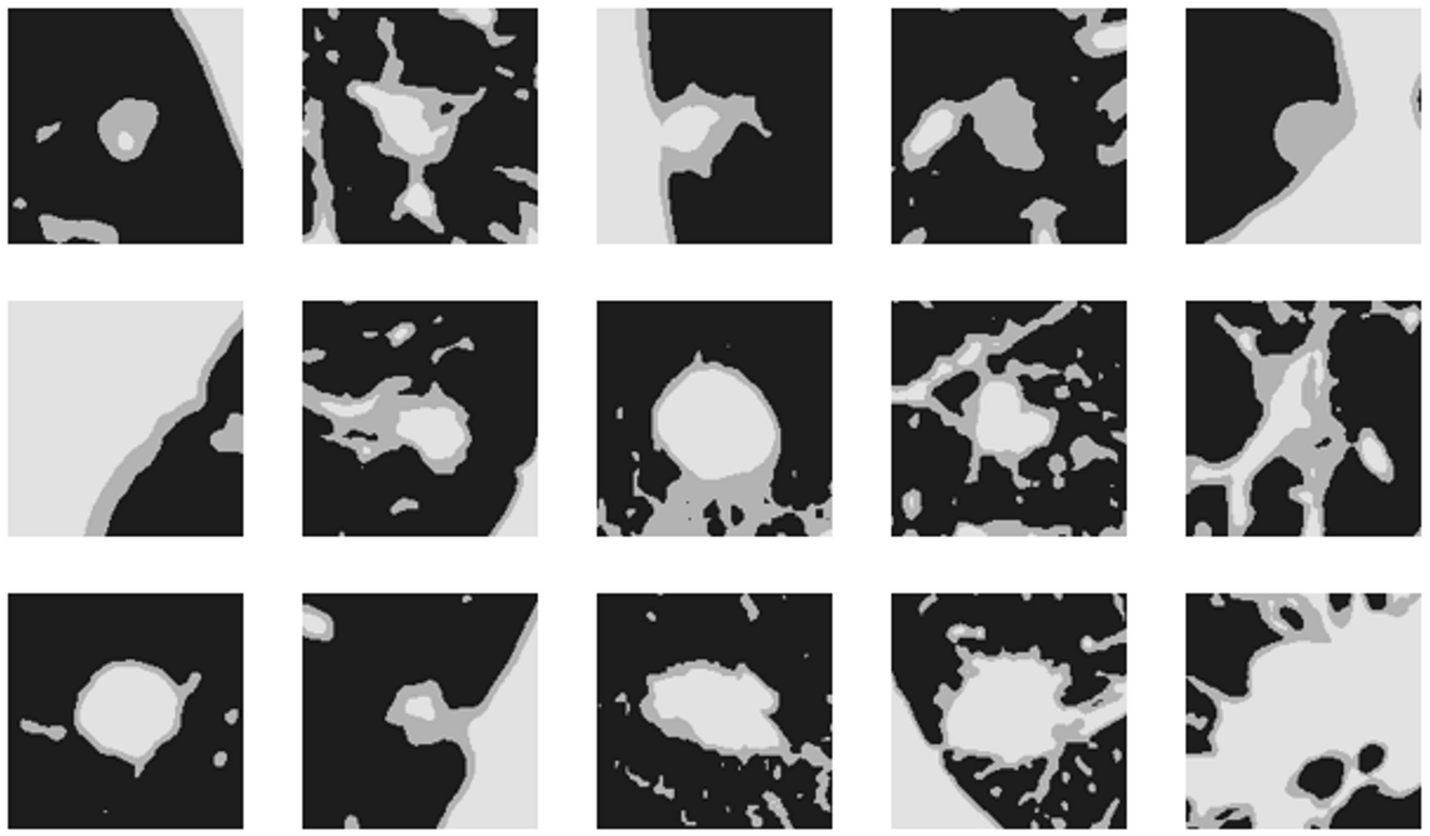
The results of the Otsu thresholding algorithm on CT data.
